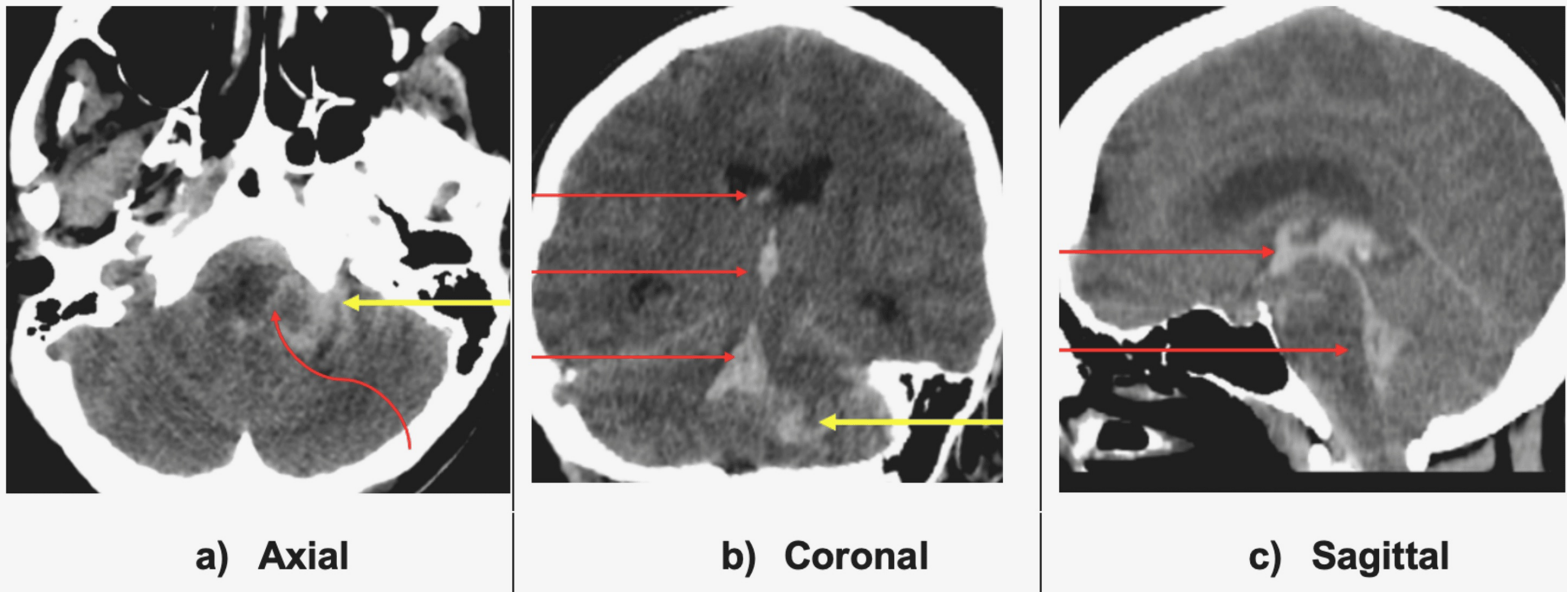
Non-contrast cranial CT scan Acute intraparenchymal left cerebellar hemorrhage (yellow arrows in A and B) with extension to the ventricles (straight red arrows in B and C) via the foramen of Luschka (curved red arrow in A) and subarachnoid cisterns.
